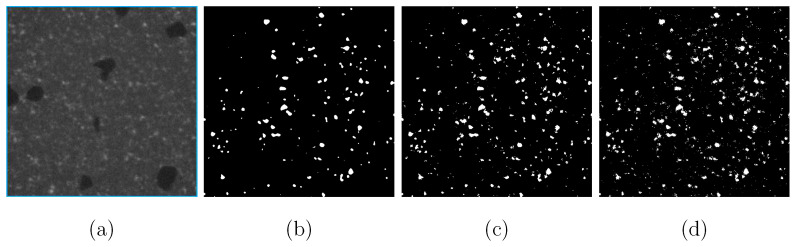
(a) An original CT image with noises after the extraction of the inscribed square out of a circle; (b–d): final denoising results of the WC phase using median, Gaussian, bilateral denoising methods [61], respectively.
